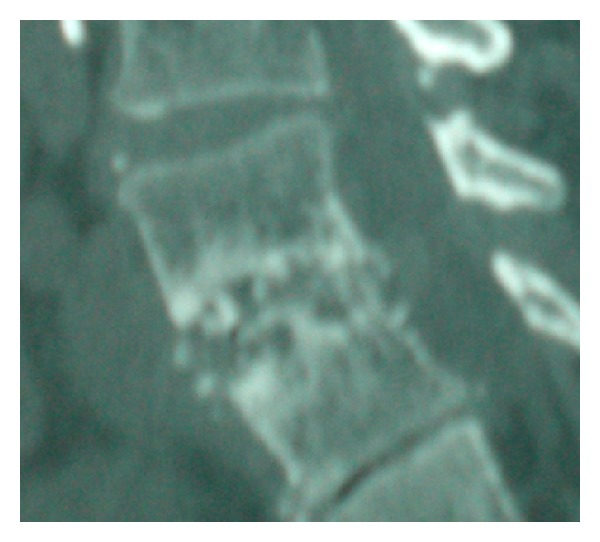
Sagittal CT scan image showing bony destruction of L4 and L5 (Case 2).
